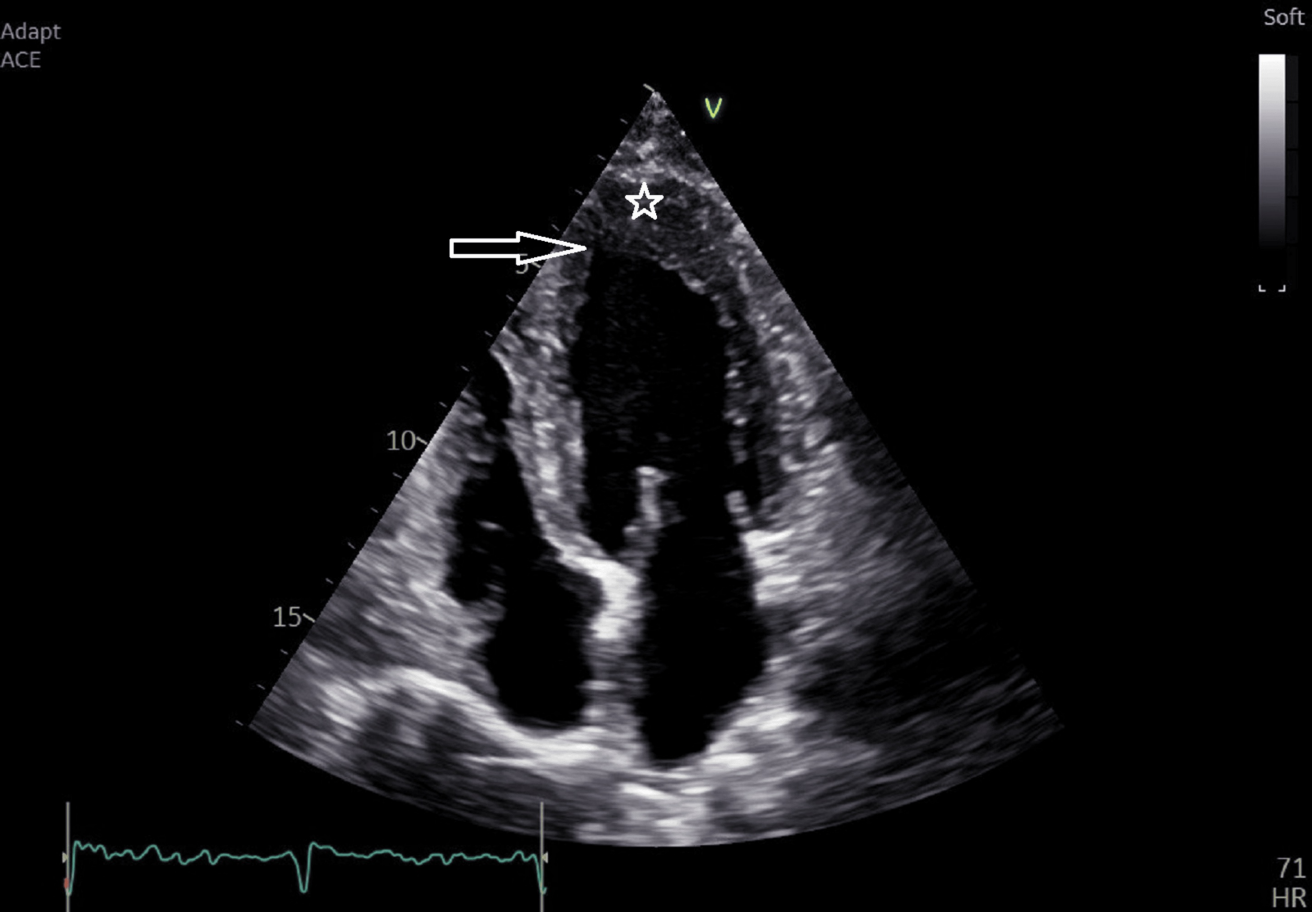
TTE showing apical hypertrophy (star) with the Ace-of-Spades configuration (arrow) TTE: trans-thoracic echocardiography
